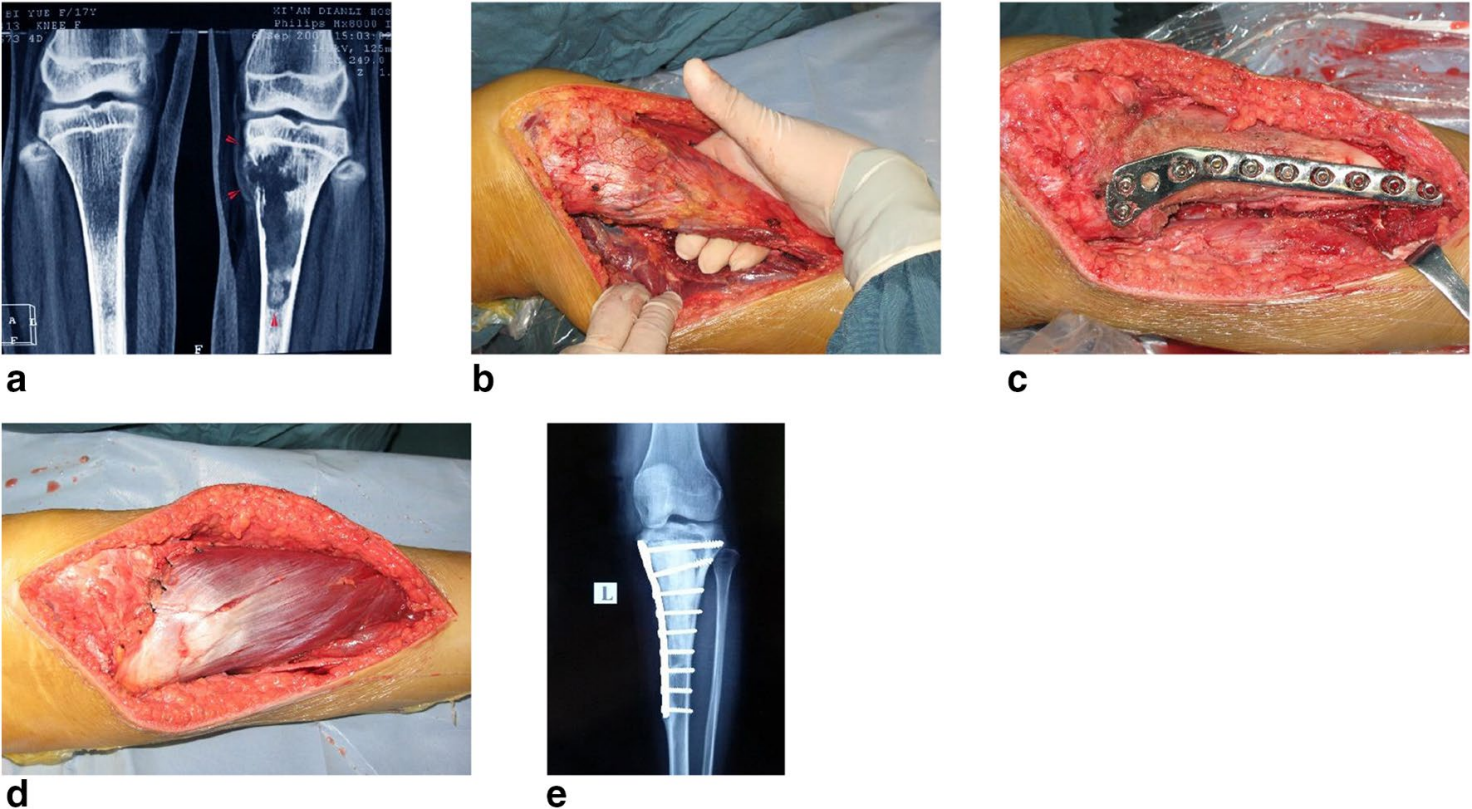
Typical procedure for osteosarcoma at proximal tibia. a X-ray film before surgery. b Isolation of tumor bone. c Give a prophylactic fixation after MWA. d Transfer the medial head of gastrocnemius muscle. e X-ray film after surgery
